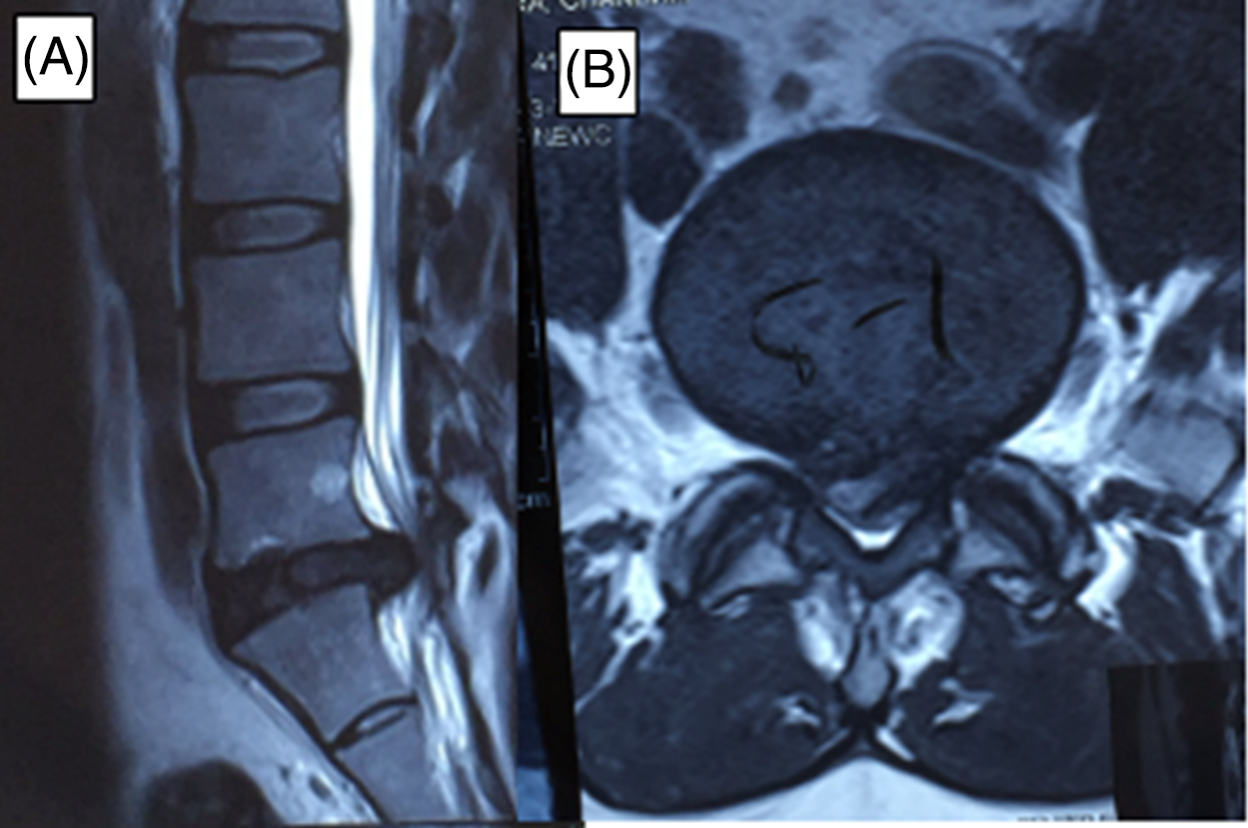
(A) Sagittal MRI showing a large disk herniation at L5‐S1. (B) Axial MRI of the L5‐S1 IVD demonstrates severe canal stenosis (grade 3) resultant from a large central disk herniation which fully compresses the thecal sac.
